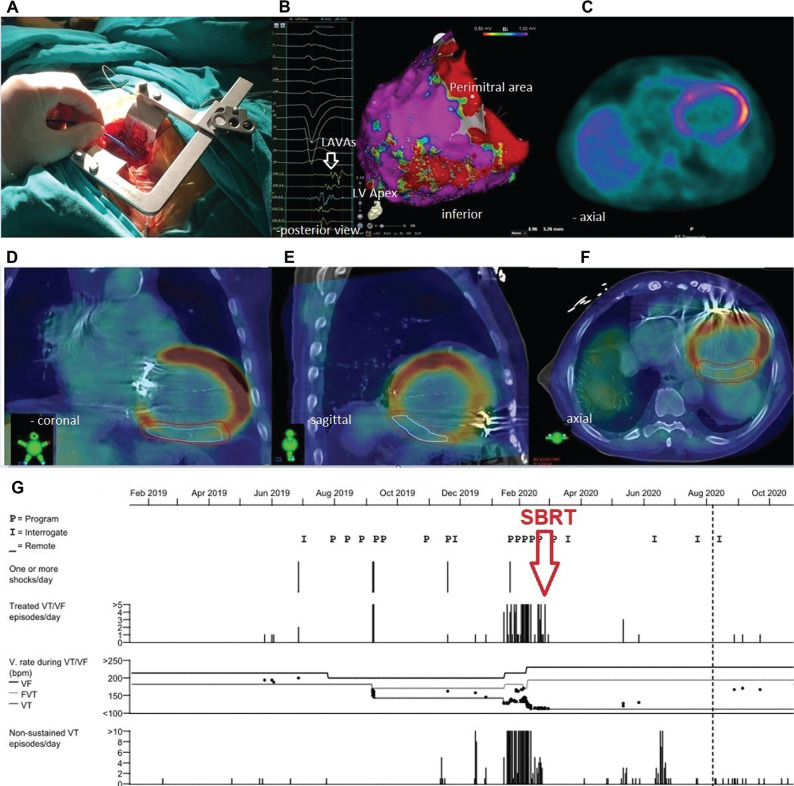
After a mini-thoracotomy (A), three-dimensional mapping (B) with images of PET-CT (C) and 4D CT (D–F) were used to detect the target substrate. The noninvasive SBRT (red arrow) was applied successfully in March 2020 (G). FVT, fascicular ventricular tachycardia; LAVAs, local abnormal ventricular activities; LV, left ventricular; SBRT, stereotactic radioablation therapy; VF, ventricular fibrillation; VT, ventricular tachycardia.
